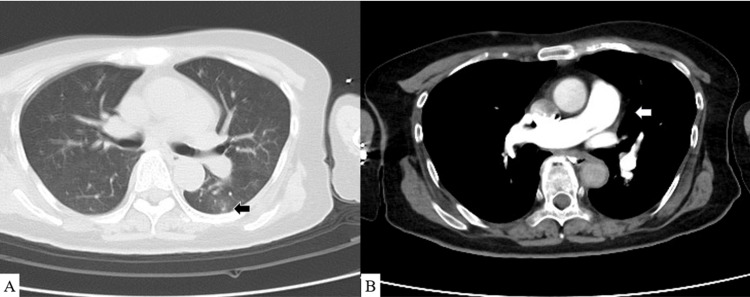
CT findings on the first day (A) Black arrow: pulmonary ground-glass opacity is observed. (B) White arrow: no contrast deficit is observed in the pulmonary artery CT: computed tomography
